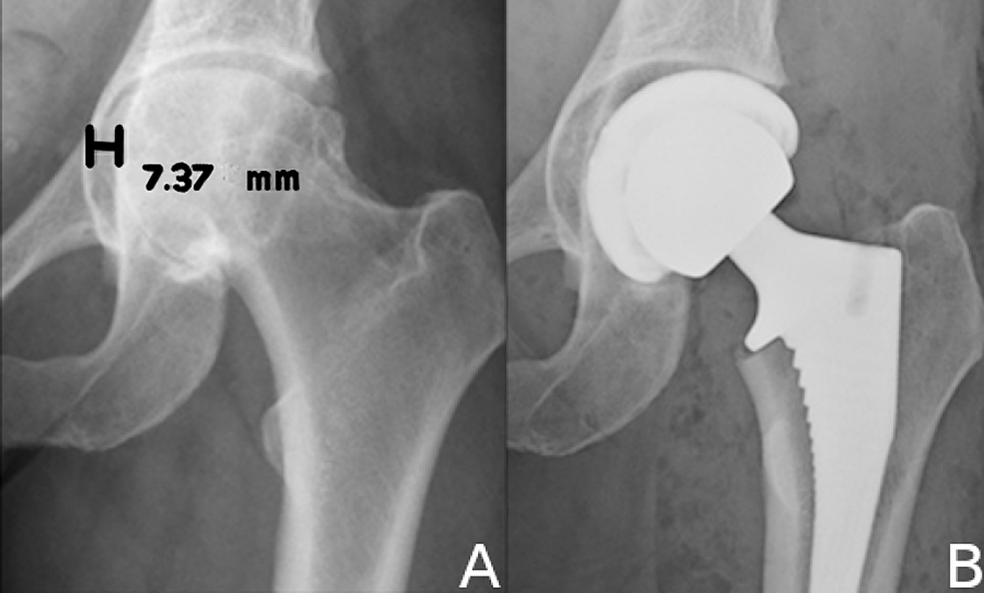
Measurement of radiographic outcomes after acetabular reconstruction. (A) Anteroposterior (AP) radiograph close-up of left hip with moderate protrusio of 7.37 mm medialization. (B) AP radiograph close-up of left hip after medial autografting and corrected center of rotation. Note the medial edge of the acetabular component is now lateral to Kohler’s line.
